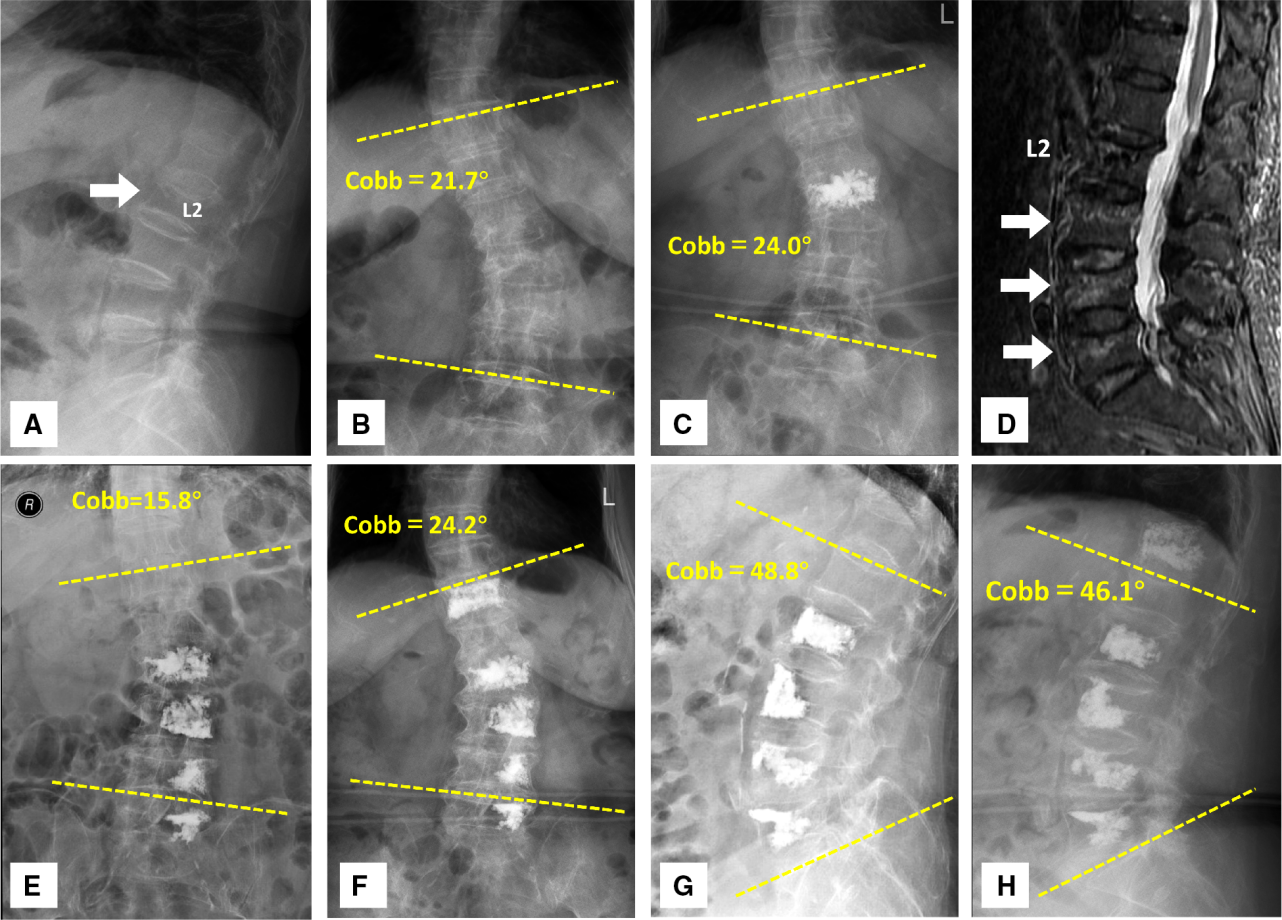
An 80-year-old woman presented with a history of osteoporotic vertebral compressive fractures and underwent multiple unilateral PVP. (A) The patient had been admitted for management of osteoporosis vertebral compressive fracture at L2 4 years ago. The white arrow indicated the fractured vertebra. (B) The preoperative radiograph showed scoliosis with a Cobb angle of 21.7°. (C) The patient was treated with PVP for L2 vertebra. The 24-h postoperative anteroposterior *x*-rays showed the cement augmentation within the L2 vertebra. (D) The patient was readmitted for management of progressive osteoporosis vertebral compressive fractures. MRI shows the signal alteration within the fractured vertebrae at L3, L4, and L5. The white arrows indicated the T2-weighted signal abnormalities in the fractured vertebrae. (E,F) A progressive Coronal Segmental Cobb angle was observed in the 24-h postoperative and 42-month follow-up anteroposterior *x*-rays of the thoracolumbar spine. A new T12 vertebral fracture had developed during the long-term follow-up and bilateral PVP were performed for the patient. (G,H) The postoperative and follow-up thoracolumbar *x*-rays demonstrated the mild changes in the sagittal segmental cobb angle.
